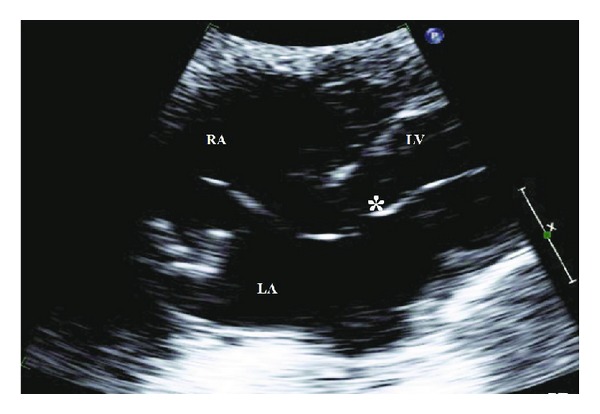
Transthoracic echocardiography, subcostal view showing the ventricular pacing lead (∗) to pass from the right atrium via interatrial septal defect to the left atrium and then via the mitral valve to the left ventricle. RA: right atrium; LA: left atrium; LV: left ventricle.
